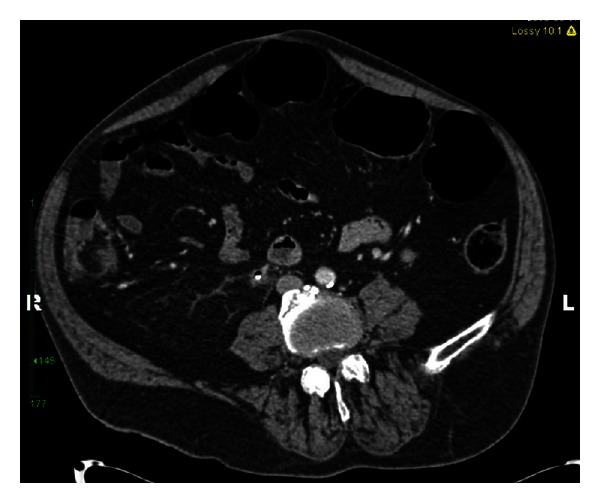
The CT image demonstrates a retained right ureteral stent and the presence of gas in the right ureter.
